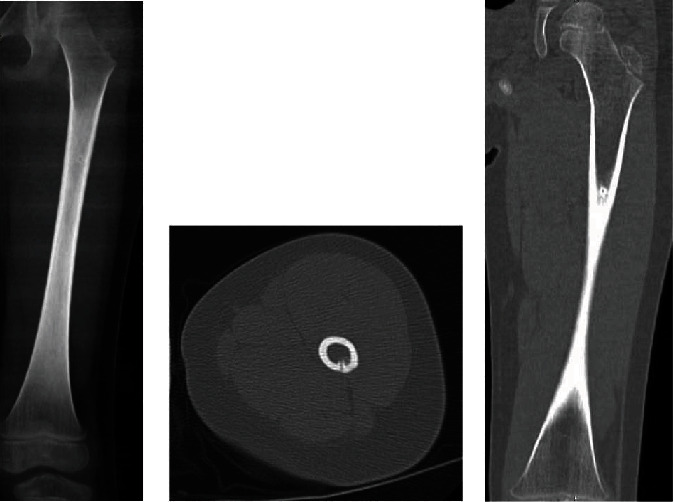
X-ray and CT images of OO 3 months prior to fracture, 2.5 months prior to RFA.
